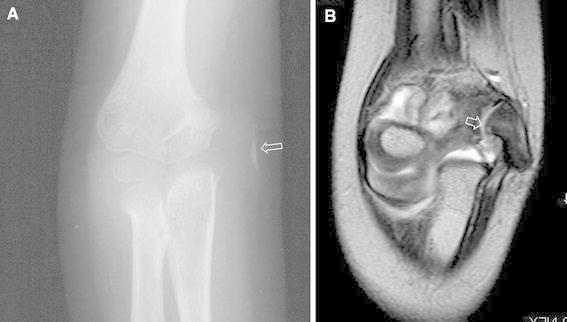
At 4 months after the initial injury, an anteroposterior radiograph showed a very thin sliver of ossification, slight narrowing of the humeroulnar joint, and mild varus alignment of the humeroulnar angle. a T2 weighted spin-echo magnetic resonance imaging (repetition time/echo time 4,000 ms/96 ms) showed complete rotation of the cartilaginous fragment (*arrow*) with trochlear defect (b)
